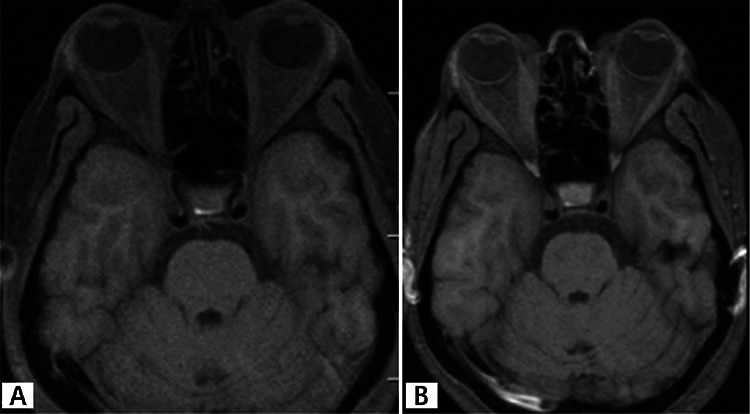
Orbital magnetic resonance imaging showing A) pretreatment fusiform thickening of the extraocular muscles and B) posttreatment regression of the extraocular muscle thickening
